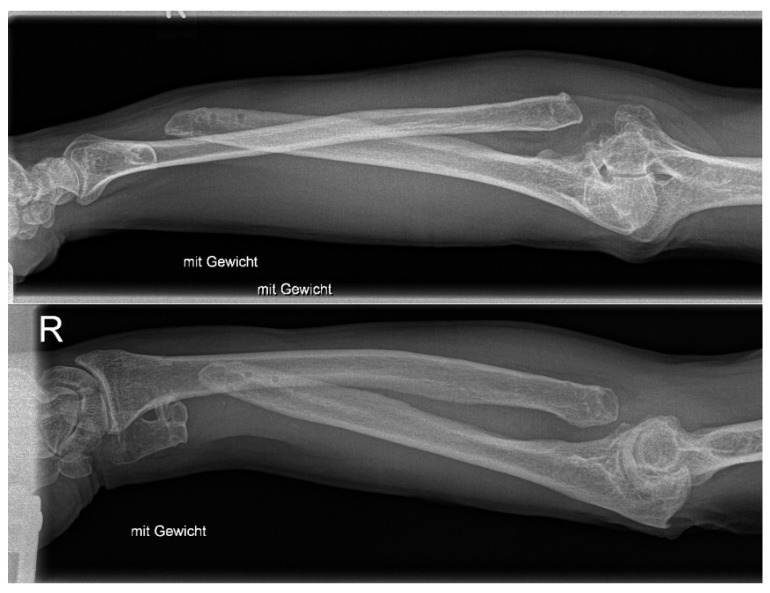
A.P. and axial X-ray of the unstable forearm under load after radial head resection, failed ulna shortening osteotomy, Sauvé-Kapandji procedure, and removal of all implants.
